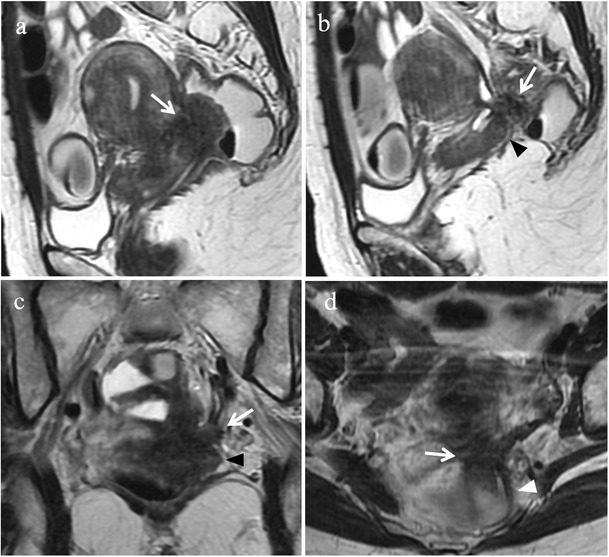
Endometriosis of the retrocervical region in a 39-year-old woman with dysmenorrhea, dyspareunia, tenesmus, catamenial dyschezia and dysuria. (a, b) Sagittal, (c) oblique coronal and (d) oblique axial T2-weighted images show a retrocervical hypointense endometriotic implant (white arrows) infiltrating the posterior uterine serosa and the anterior rectal wall, extending to the left vaginal fornix (black arrowheads in b and c) and to the left uterosacral ligament (white arrowhead in d)
